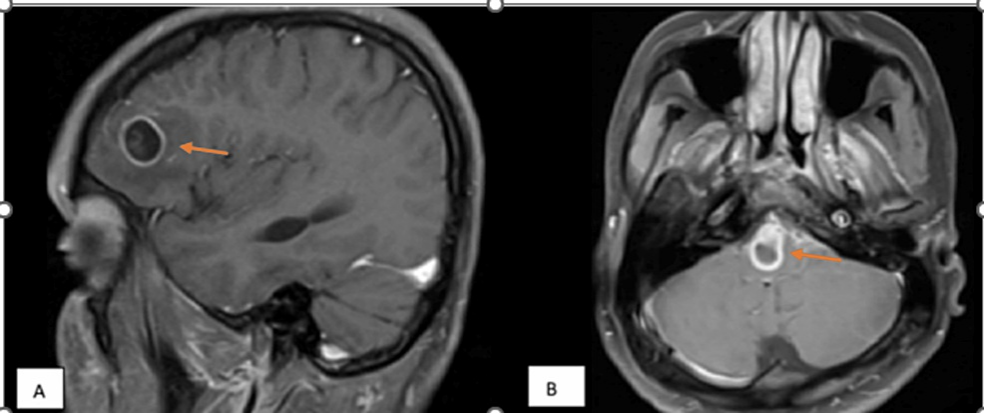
Post-contrast magnetic resonance images of neurocysticercosis and tuberculoma cases (A) Case of neurocysticercosis: MRI T1-weighted post-contrast image showing thin ring enhancement of the lesion in the left frontal lobe (orange arrow). (B) Case of tuberculoma: MRI T1-weighted post-contrast image showing thick irregular enhancement of the lesion in the pons (orange arrow).
